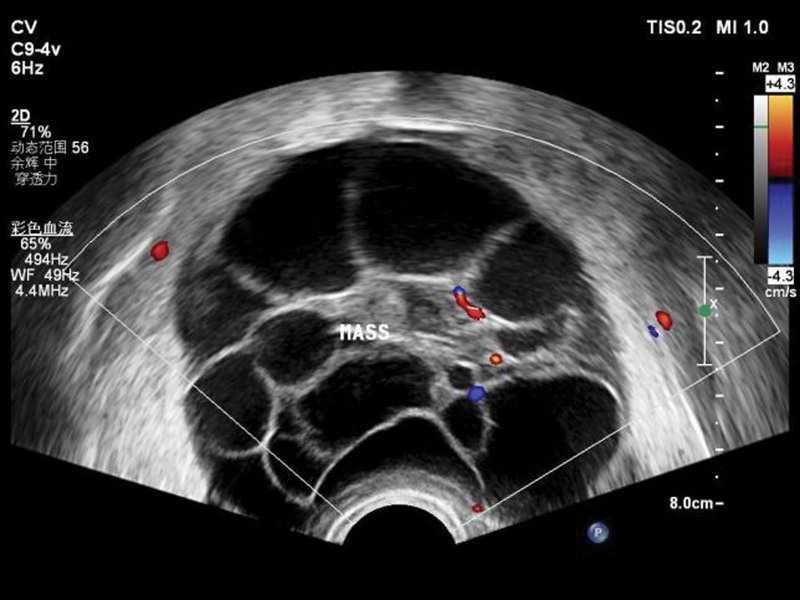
Gynecological ultrasound showed a cystic mass on the right posterior of the uterus, with irregular shape and multiple partitions.
